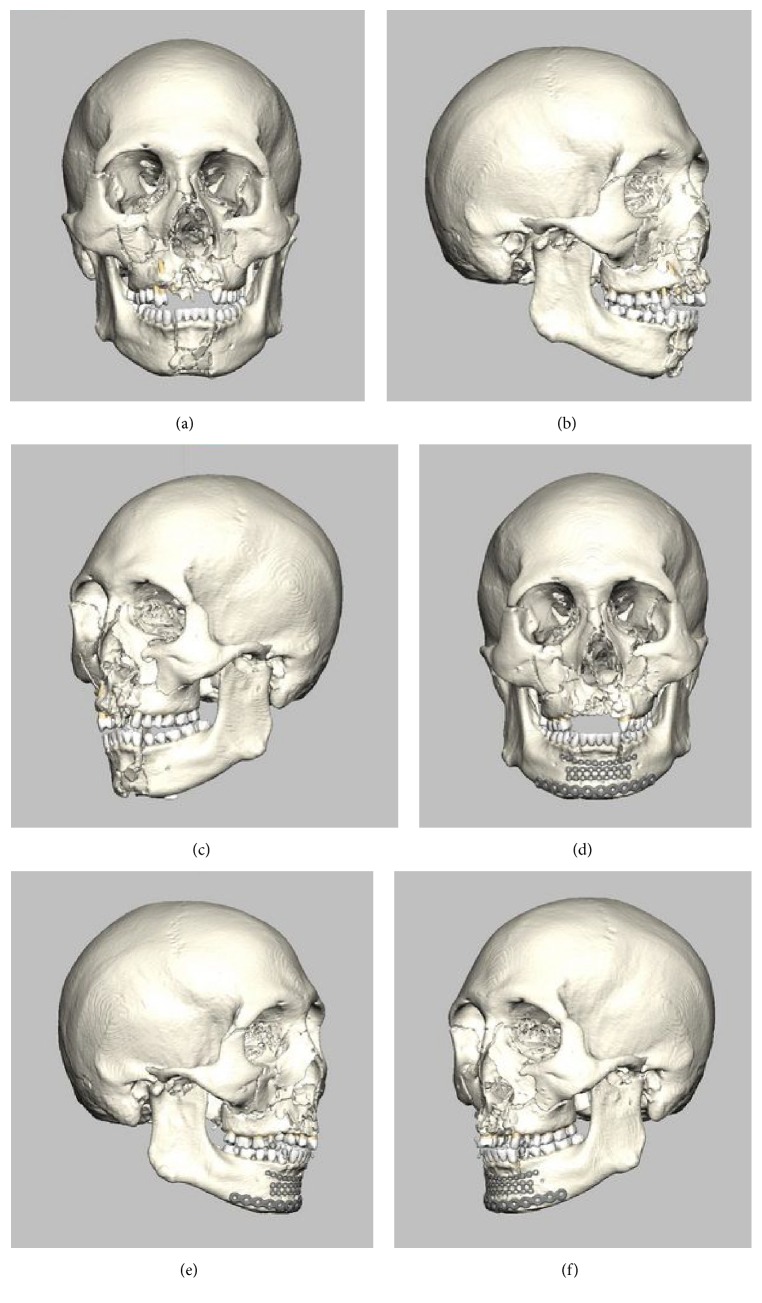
Images from preoperative and postoperative 3D-CT. (a)–(c) showed that bilateral condylar and mandibular fractures, LeFort II-type fracture, and blow-out fracture of orbit are apparent. (d)–(f) showed that mandibular fracture was fixed by titanium plates and LeFort II-type fracture and blow-out fracture of the orbit were fixed by absorbable plates. (a) Preoperative frontal view. (b) Preoperative lateral view of the right. (c) Preoperative lateral view of the left. (d) Postoperative frontal view. (e) Postoperative lateral view of the right. (f) Postoperative lateral view of the left.
